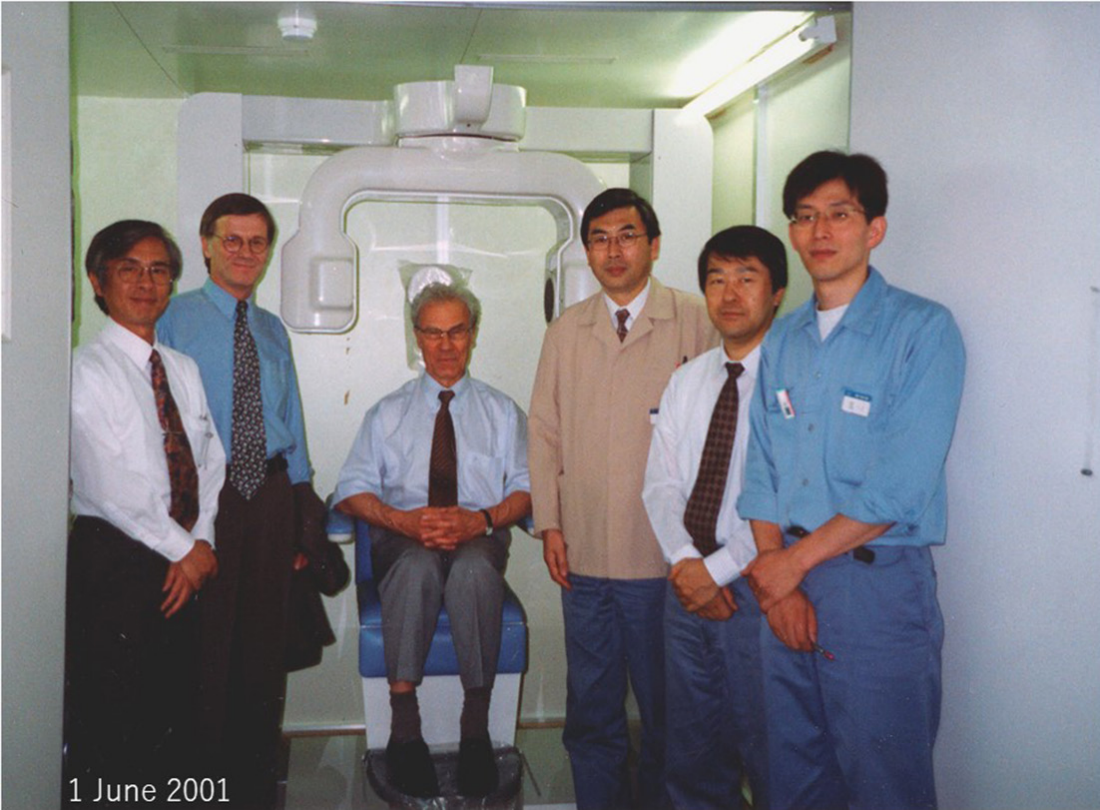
Completed local cone beam CT Center: Professor E. Tammisalo named the system “*Accuitomo*” alongside the development team of J. Morita Mfg. Co. on June 1, 2001.
